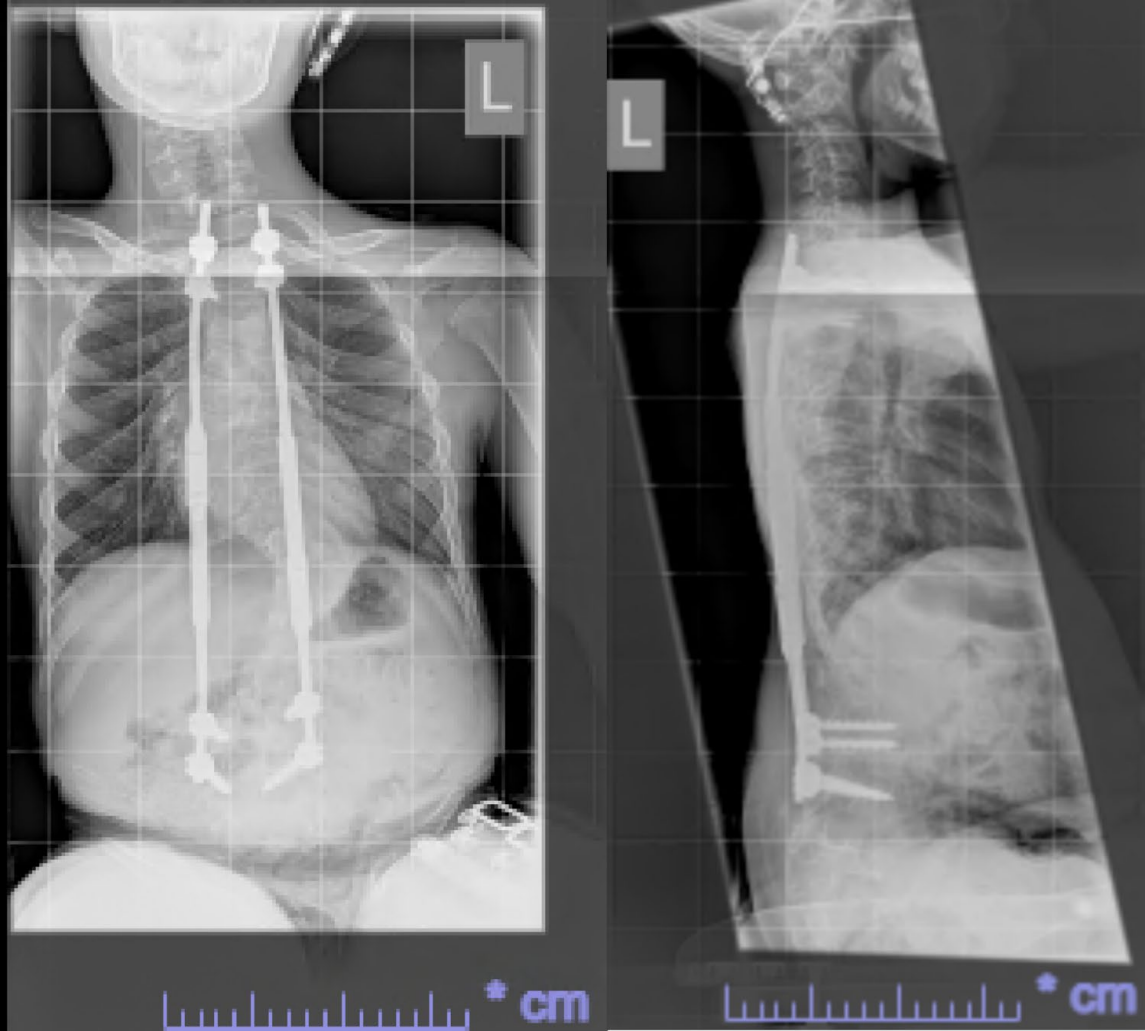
Post operative X-ray after implantation of MAGEC rods, aged 10
